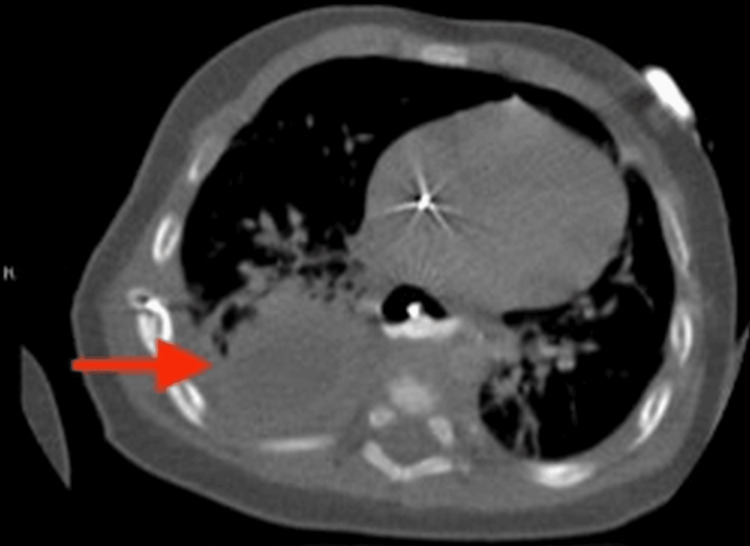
Large abscess seen on computed tomography, drained by interventional radiology.
